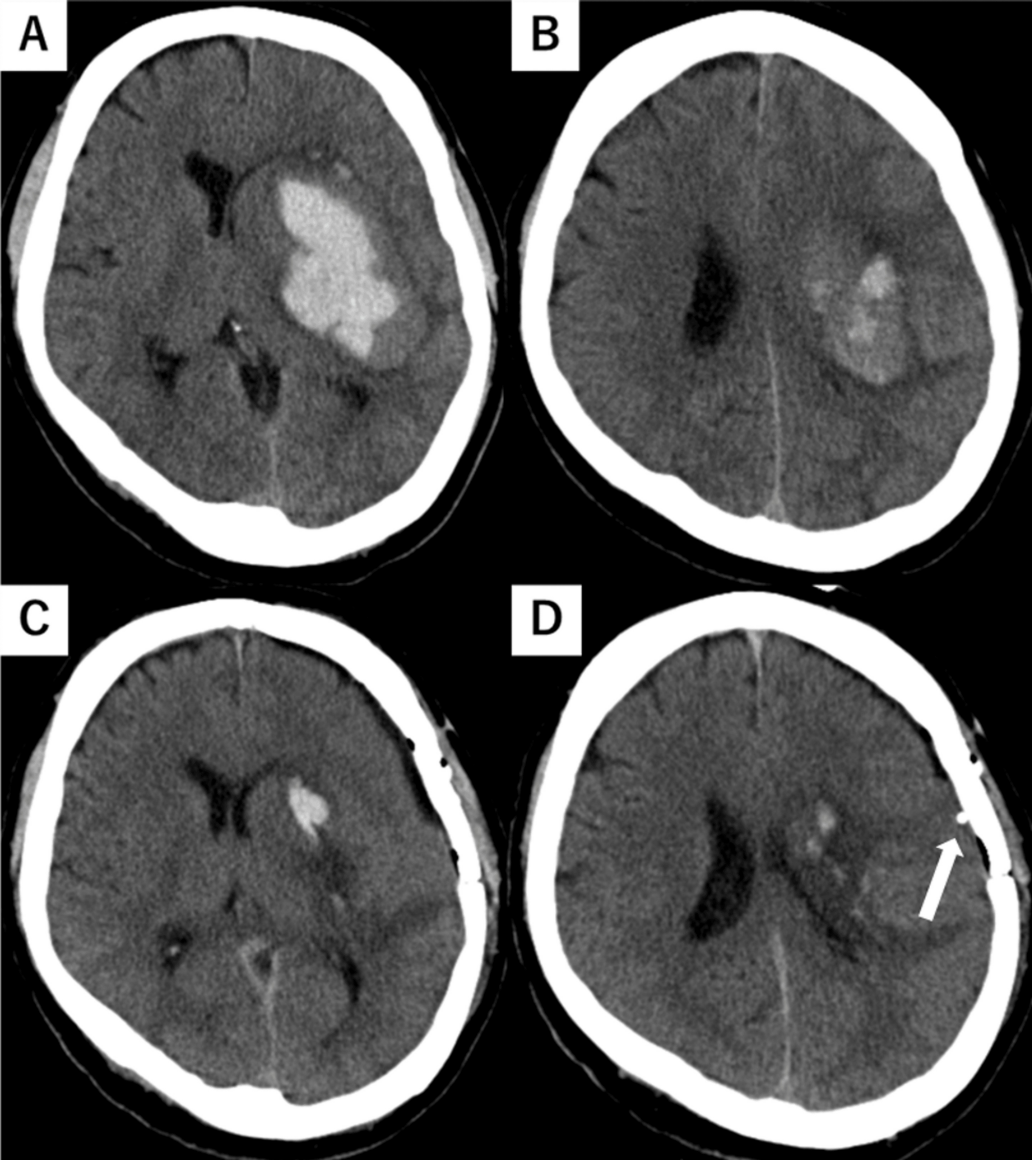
Preoperative and postoperative brain CT. (A–B) Preoperative brain CT. The hematoma is located in the putamen, and measures approximately 60 mL and is associated with a mild midline shift and obscuration of the left sulcus. The hematoma was measured as 6.7 cm (length) × 5.5 cm (width) × 3.3 cm (height). (C–D) Postoperative brain CT. The hematoma in the putamen has been removed, while a hematoma remains in the left caudate nucleus. The midline shift has improved; however, the obscuration of the left sulcus persists. ICP devices(probe) are indicated by white arrows. CT, computed tomography.
